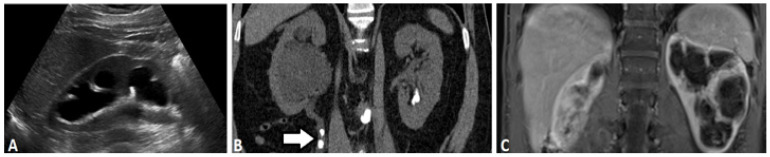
Different imaging modalities of kidney including right renal ultrasound (A), coronal non-contrast CT (B) and contrast enhanced MRI (C) demonstrating unilateral hydronephrosis. Note the presence of obstructing proximal uretal stones in the CT (arrow).
